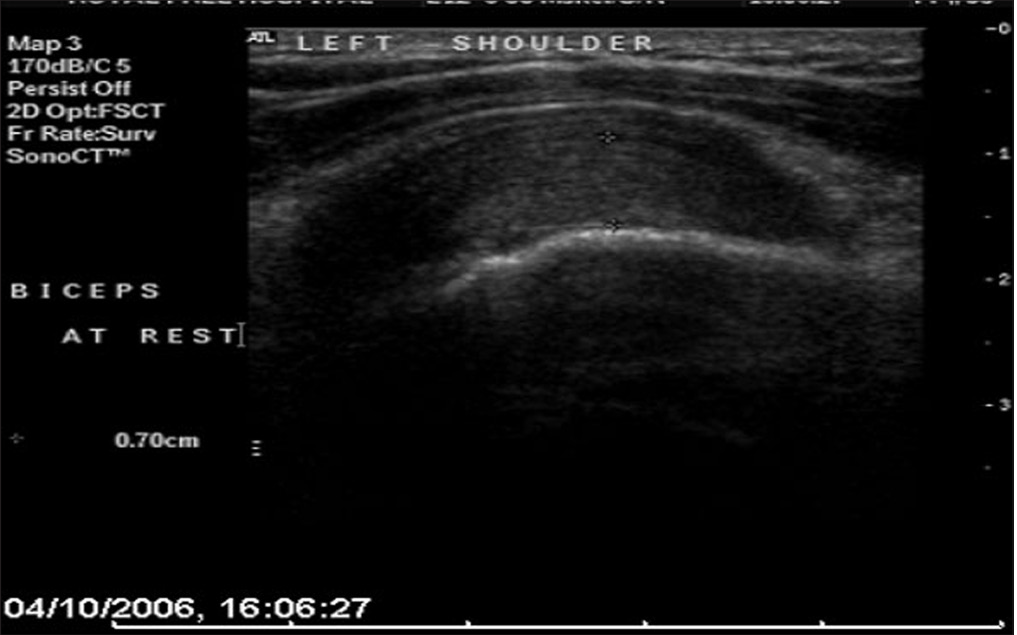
Ultrasound image of a longitudinal view of the LHB at rest
